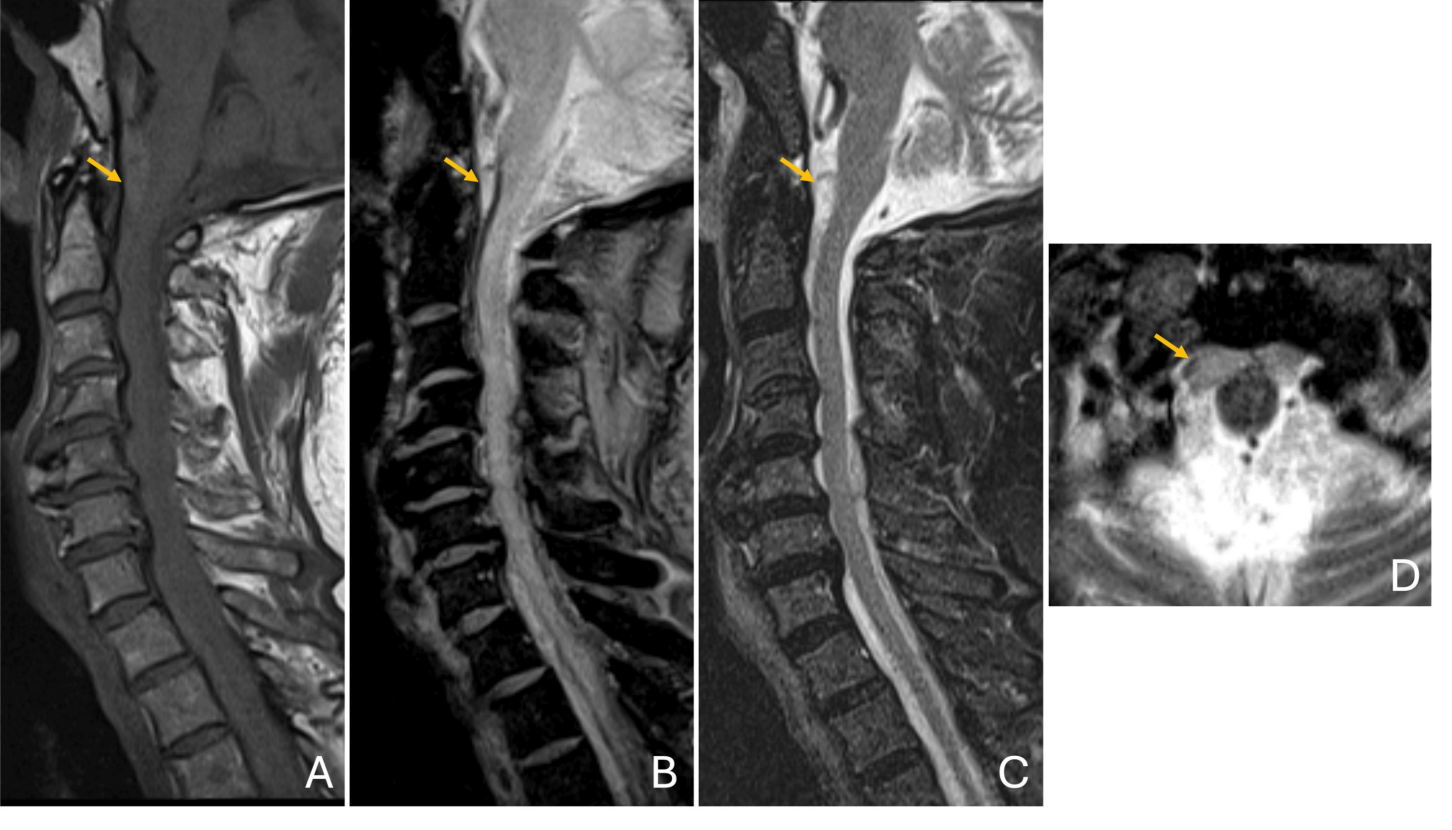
Cervical MRI. Large hematoma extending from the pre-pontine cistern, predominantly anterior to the spinal cord, with involvement throughout the entire cervical segment and the upper thoracic region, partially captured within the field of view. The hematoma exhibited isointense signal on T1-weighted imaging (A), blooming on T2* (B), and hyperintensity on STIR (C), blending with the CSF signal but demonstrating hypointense signal on T2, particularly evident at the level of the cisterna magna (D, yellow arrow). MRI, magnetic resonance imaging; CSF, cerebrospinal fluid; STIR, short tau inversion recovery.
